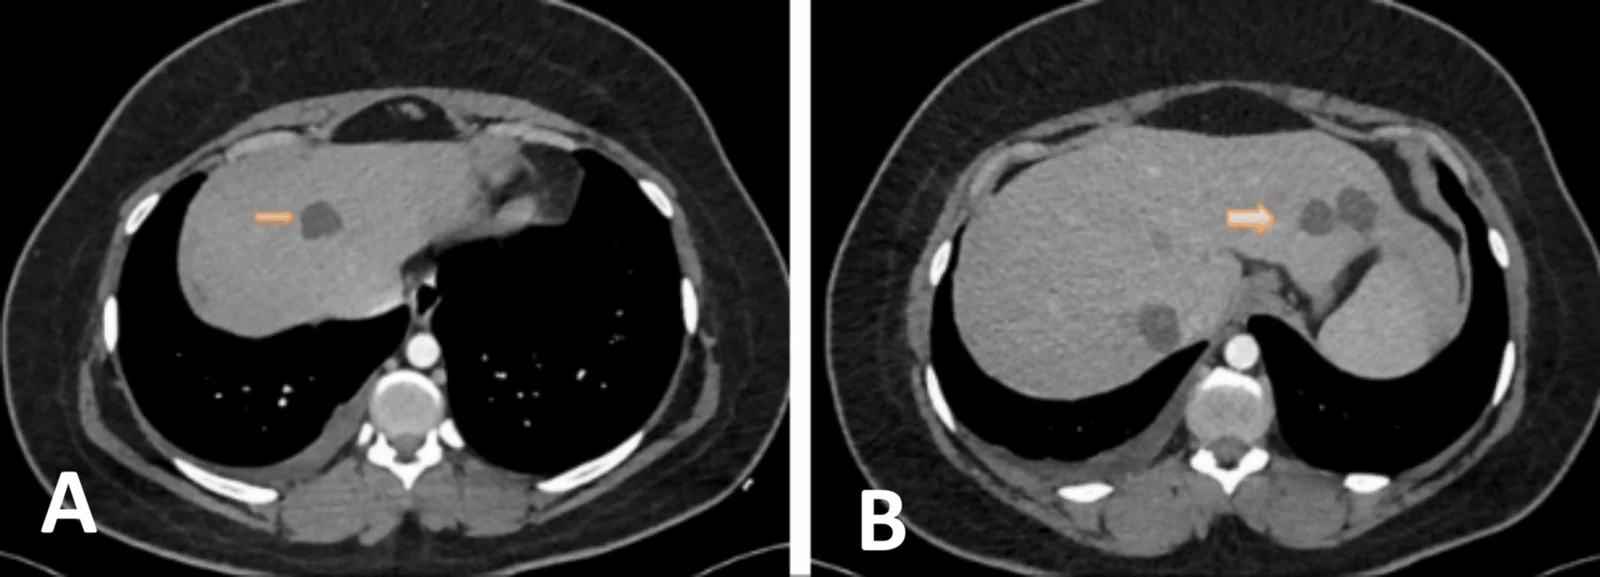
CT scan of the liver on admission: (A, B) cross-sectional images of the liver showing hypodense lesions The arrows indicate hypodense hepatic lesions representing cystic involvement by *Echinococcus*.
